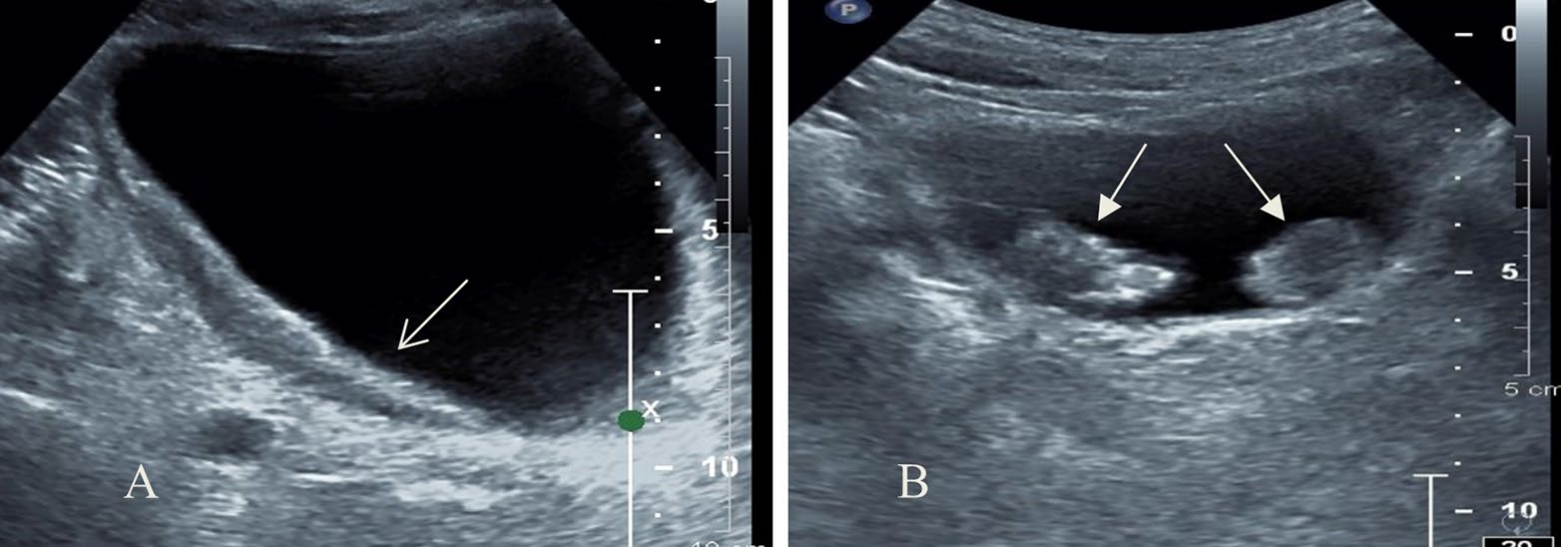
Lesion morphology. (A) Diffuse thickening of bladder wall can be seen by two-dimensional ultrasound (arrow). (B) Multiple hypoechoic nodules on the bladder wall protrude to the bladder (arrow)
